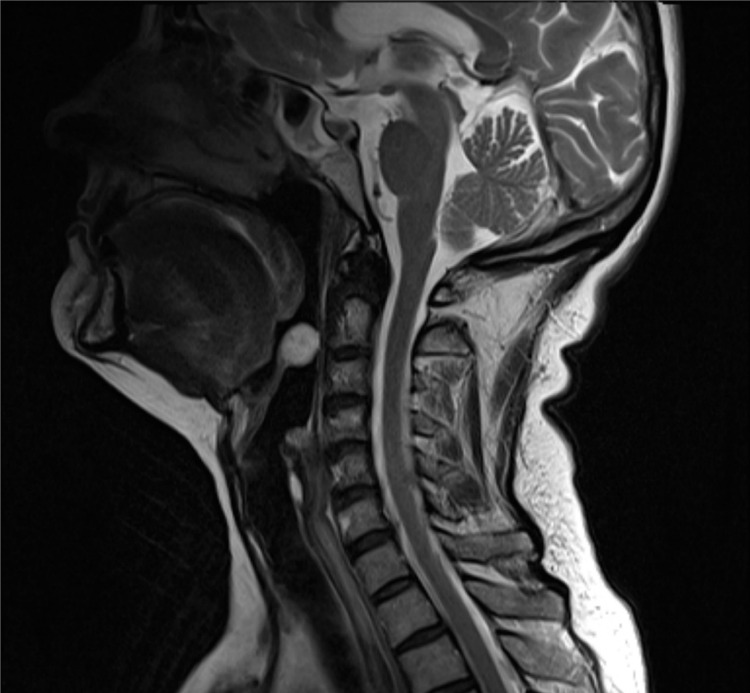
T2 weighted sagittal magnetic resonance imaging showing a smooth, well defined mass arising from the tip of the epiglottis
